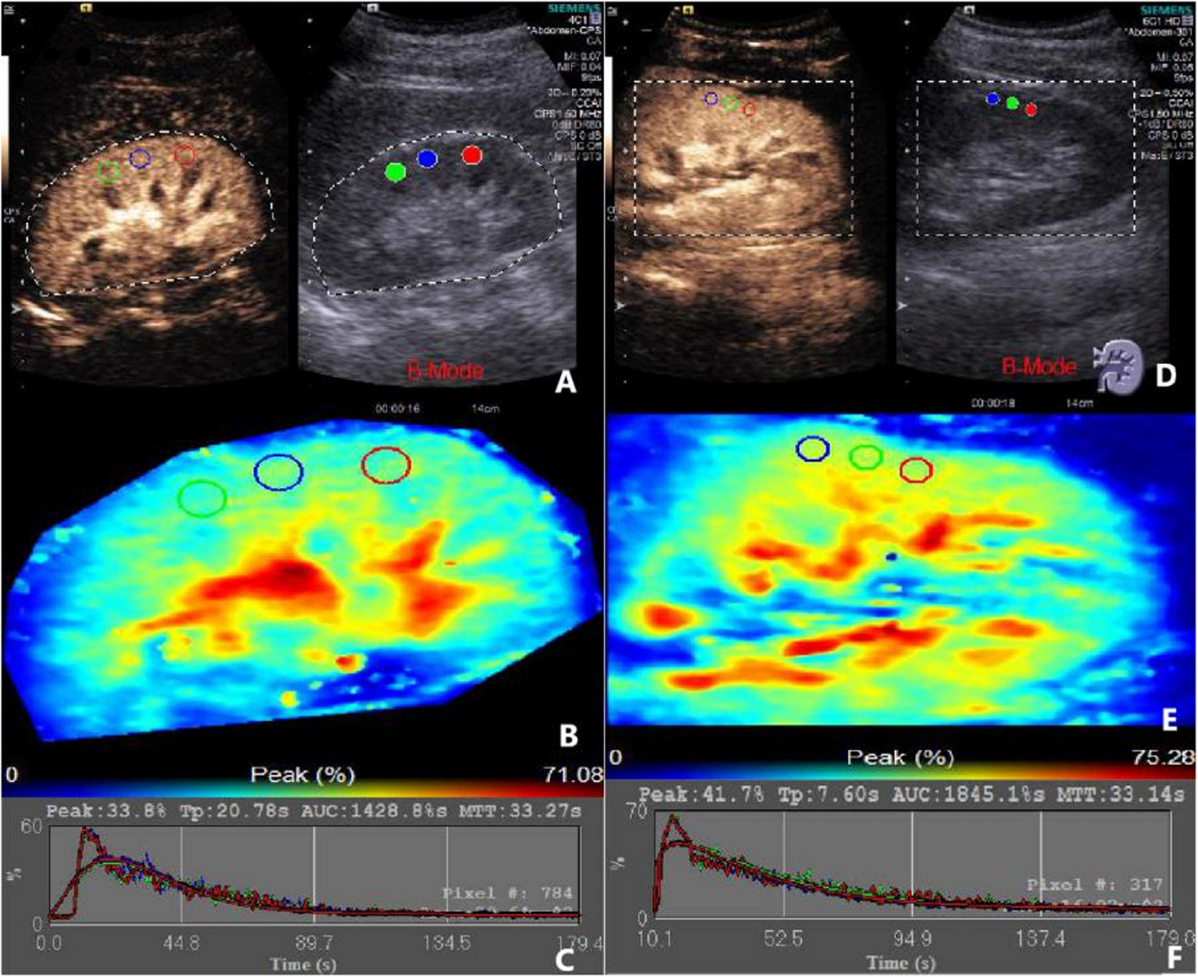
Left renal angiography by contrast ultrasound. a, b and c showed the image of left renal angiography of a 58-year-old man with DN and body mass index of 24.5 in stage CKD grade 1. d, e and f showed the image of left renal angiography of a 61-year-old man of NDRD with body mass index of 24.7 in CKD grade 1
